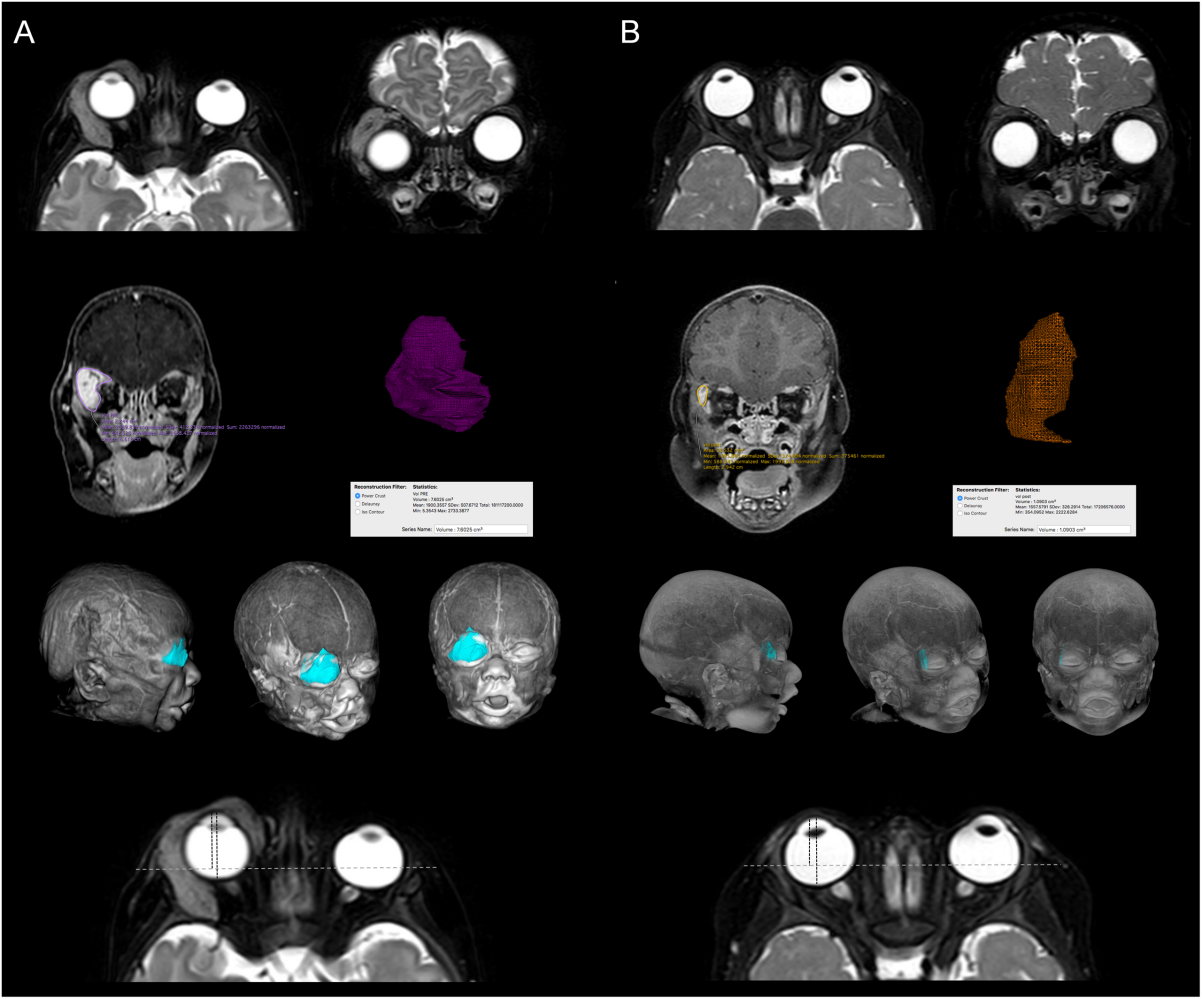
Comparison of MRI images before (A) and after treatment (B). Axial and Coronal T2 SPIR MR Images show almost complete resolution of the right periorbital hemangioma; note the resolution of the proptosis due to the reduction of the compression of the eyeball. Segmentation, volume calculation and 3D representation of the periorbital hemangioma and the residual portion after treatment are showed. Resolution of exophthalmos is also detected (Cabanis oculo-orbital index 84% vs 69%).
